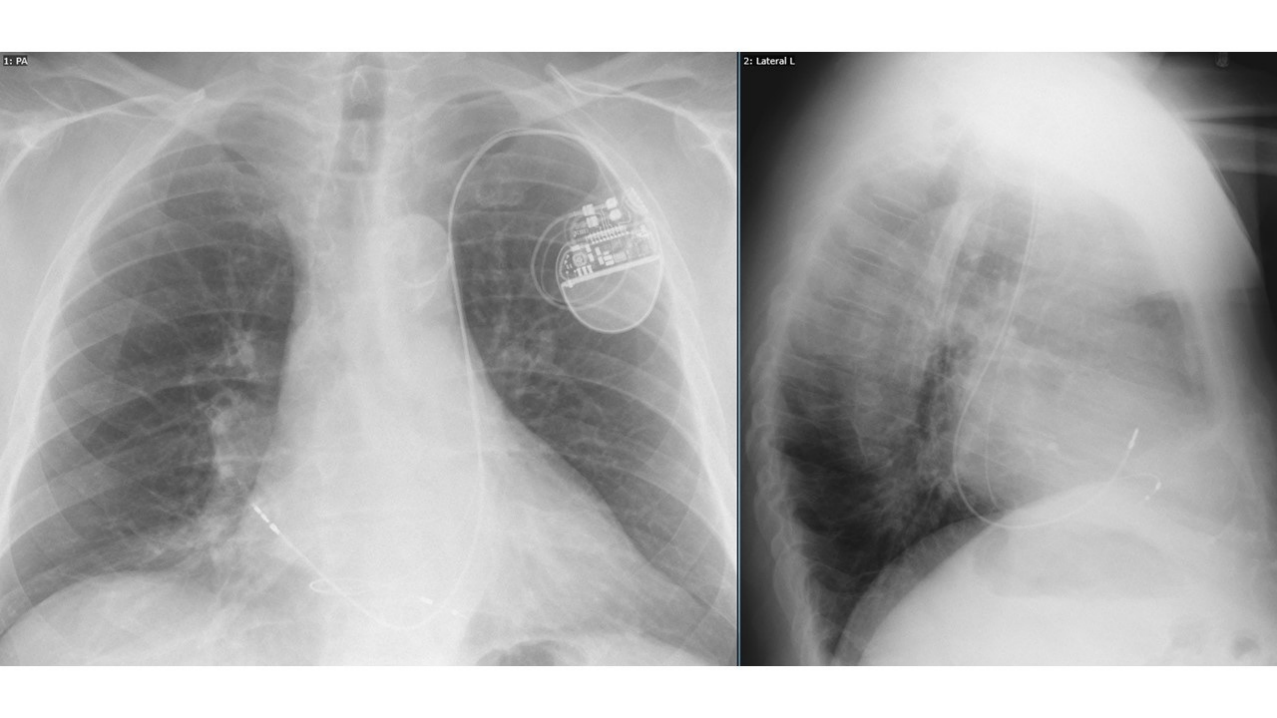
Post-procedure chest x-ray
